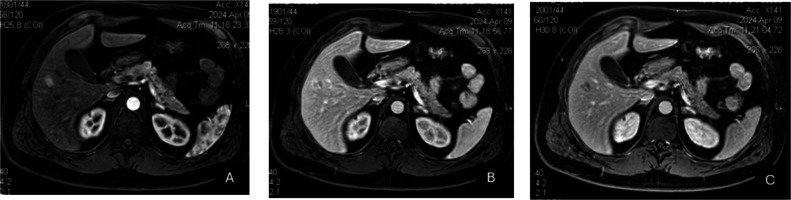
MRI aspects in arterial (A), venous (B) and tardive (C) phases
